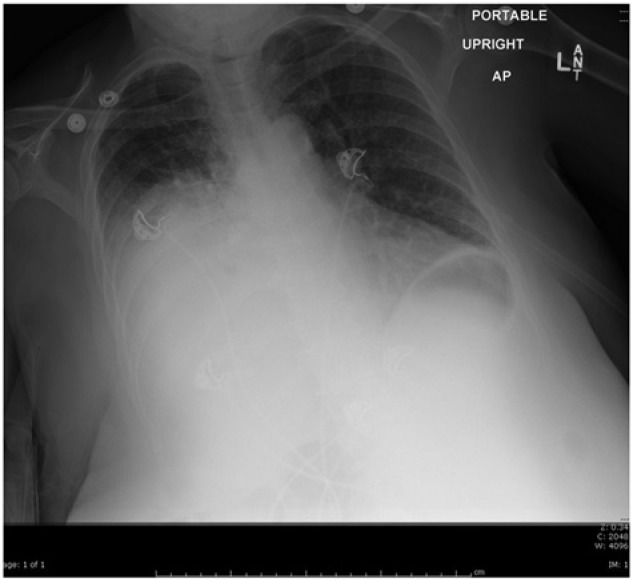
Chest X-ray presenting opacity in the right hemithorax.
